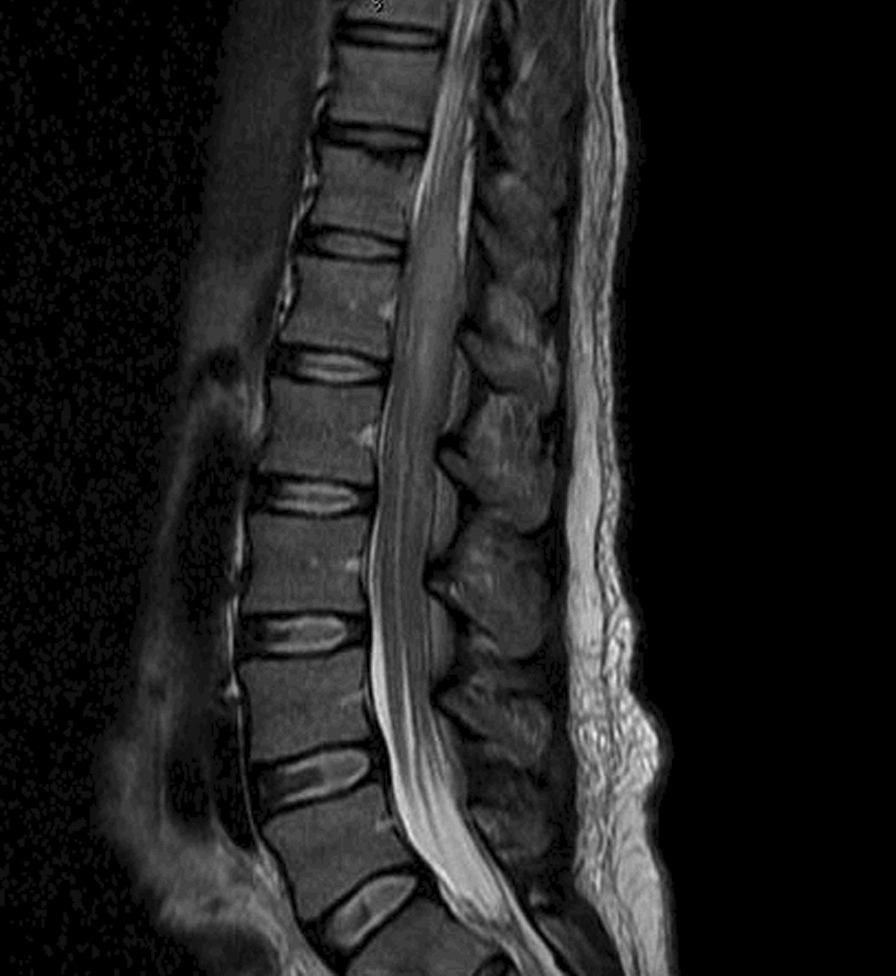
MRI Lumbar-Thoracic Spine with contrast showing large lumbar intradural enhancing mass involving diffusely the nerve roots of cauda equina and conis medullaris. The edema extends from T10 to the conus medullaris. The tumoral component extends to the lumbar neural foramina to the dorsal ganglia
